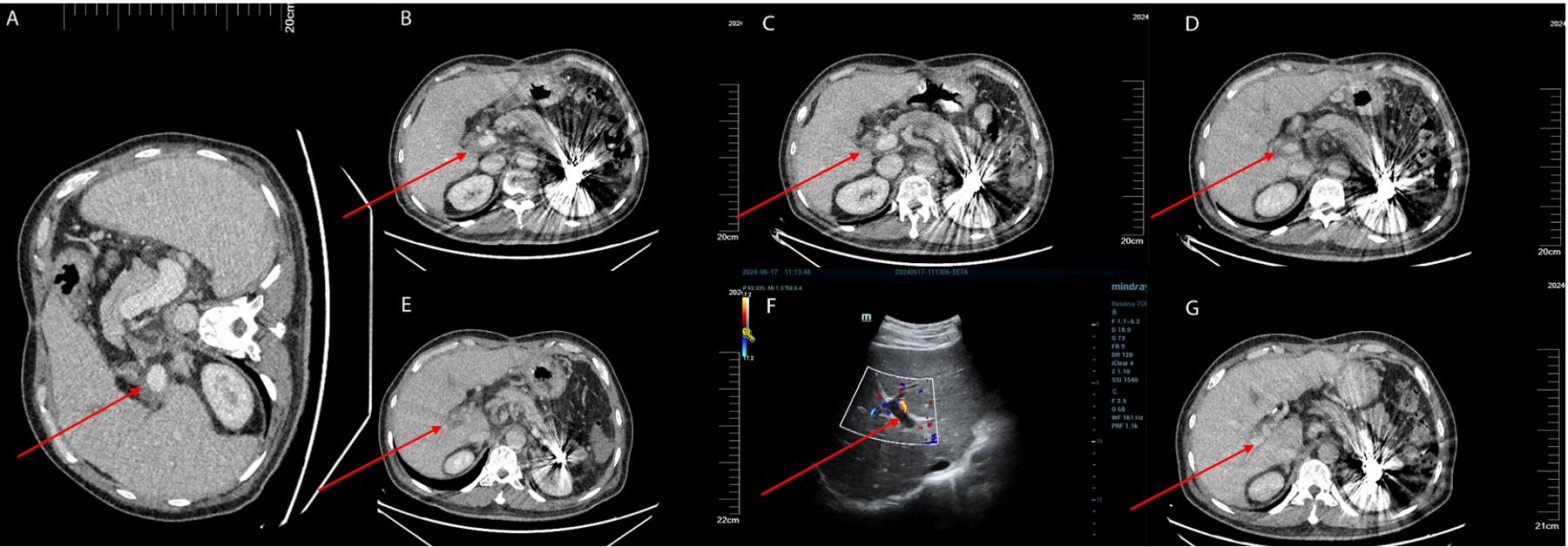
(A) The patient underwent a follow-up enhanced abdominal CT scan on January 11, 2024, prior to splenectomy, which showed no thrombus in the portal vein. (B) The patient underwent an enhanced abdominal CT scan on March 11, 2024, with the red arrows indicating the location of the portal vein thrombosis. (C) The patient had a follow-up enhanced abdominal CT scan on April 2, 2024, which showed that the extent of the thrombosis had reduced compared to the previous scan. The red arrows indicate the location of the portal vein thrombosis. (D) The patient underwent a follow-up enhanced abdominal CT scan on June 3, 2024, which revealed a new thrombus in the right branch of the portal vein. The red arrows indicate the location of the portal vein thrombosis. (E) The patient had a follow-up enhanced abdominal CT scan on June 10, 2024, which showed an increase in the extent of the portal vein thrombosis compared to the previous scan. The red arrows indicate the location of the portal vein thrombosis. (F) The patient underwent a follow-up liver Doppler ultrasound on June 17, 2024, which still showed the presence of a portal vein thrombosis. The thrombus had not completely occluded the portal vein. The red arrows indicate the location of the portal vein thrombosis. (G) The patient underwent a follow-up enhanced abdominal CT scan on June 30, 2024, which demonstrated a decrease in the size of the portal vein thrombus compared to the previous scan. The red arrows indicate the location of the portal vein thrombosis.
